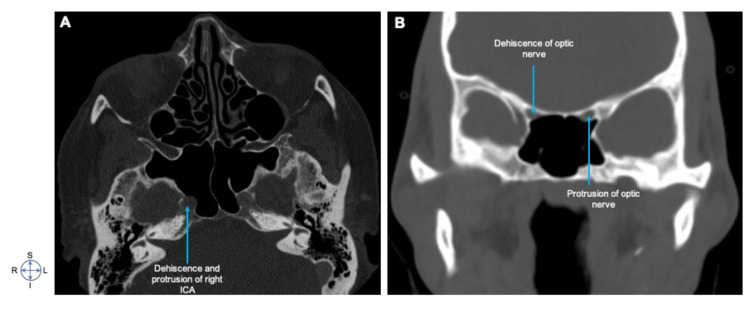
Dehiscence and protrusion of sphenoid sinus in relationship to internal carotid artery (ICA) and optic nerve. Axial CT image (A) showing dehiscence and protrusion of right ICA, (B) showing dehiscence of optic nerve (R) and protrusion of optic nerve (L). Note the differential protrusion and dehiscence in Ethiopian population. Image orientation: S, superior; I, inferior; R, right side; L, left side.
